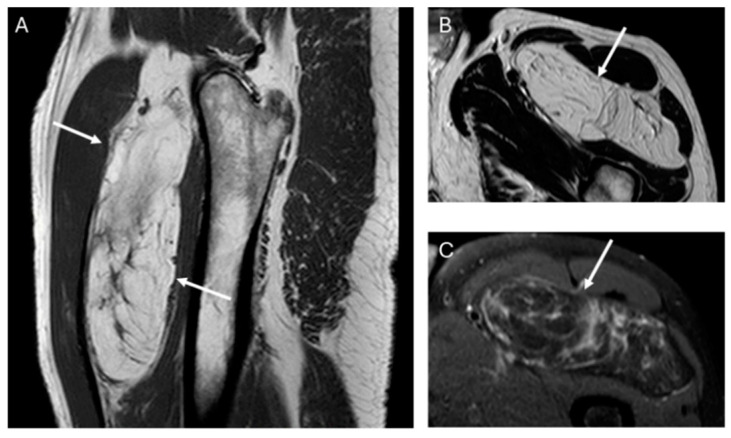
Sagittal T1W (A) and axial T2W (B) and DP SPAIR (C) MR images with a heterogeneous lipomatous mass (arrows) in the anterior compartment of the left thigh. The final diagnosis was atypical lipomatous tumor (ALT) confirmed as MDM2 positive.
